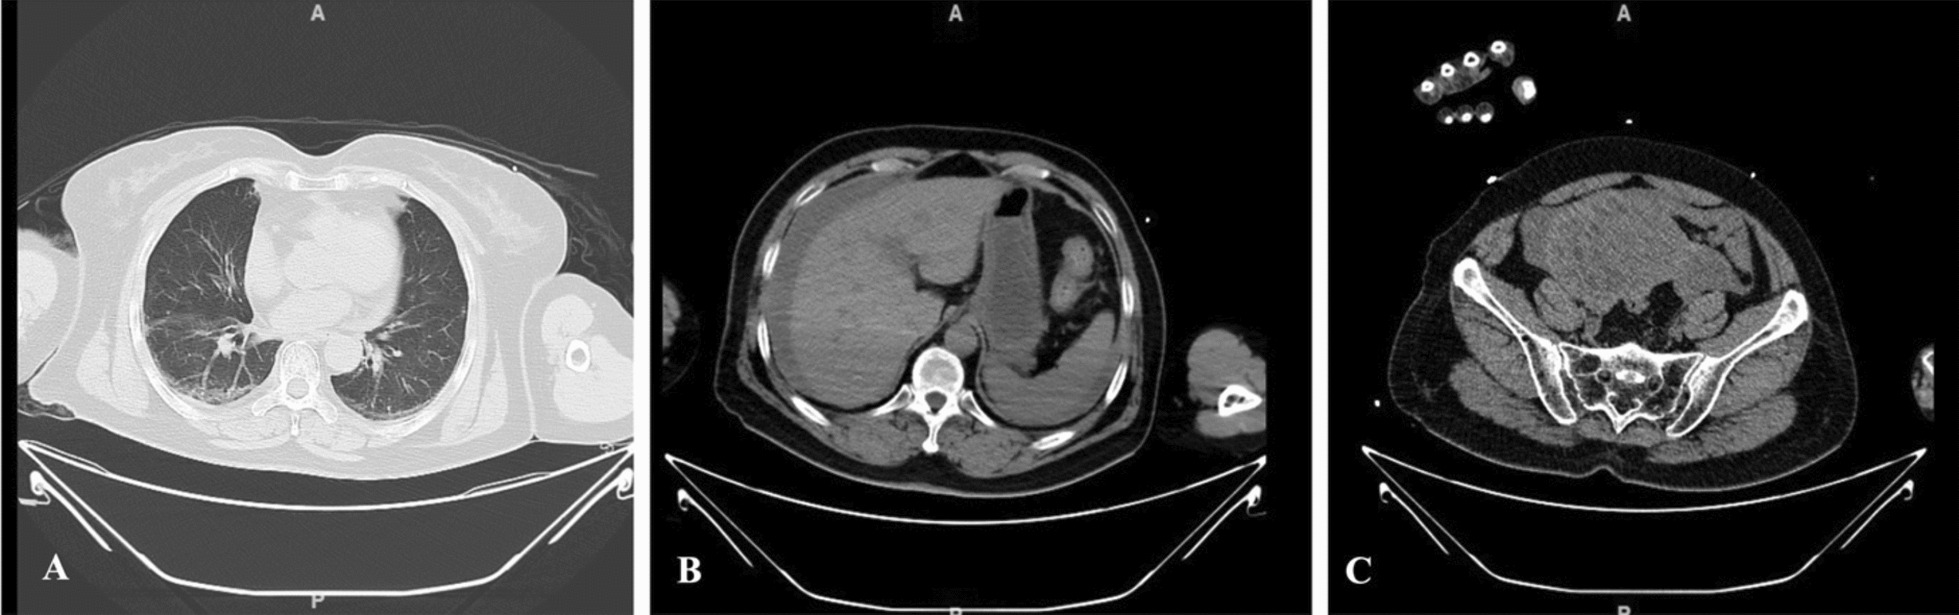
Preoperative CT scan. A Pulmonary CT scan showing subpleural arc-shaped opacities in both lungs; Small amounts of fluid in both pleural cavities; Small amounts of fluid in the pericardial sac. B Abdominal CT scan showing fluid accumulation around the liver and spleen. C Pelvic CT scan showing bilateral solid masses in the adnexal regions, possibly with associated bleeding; Small amounts of fluid in the pelvic cavity
